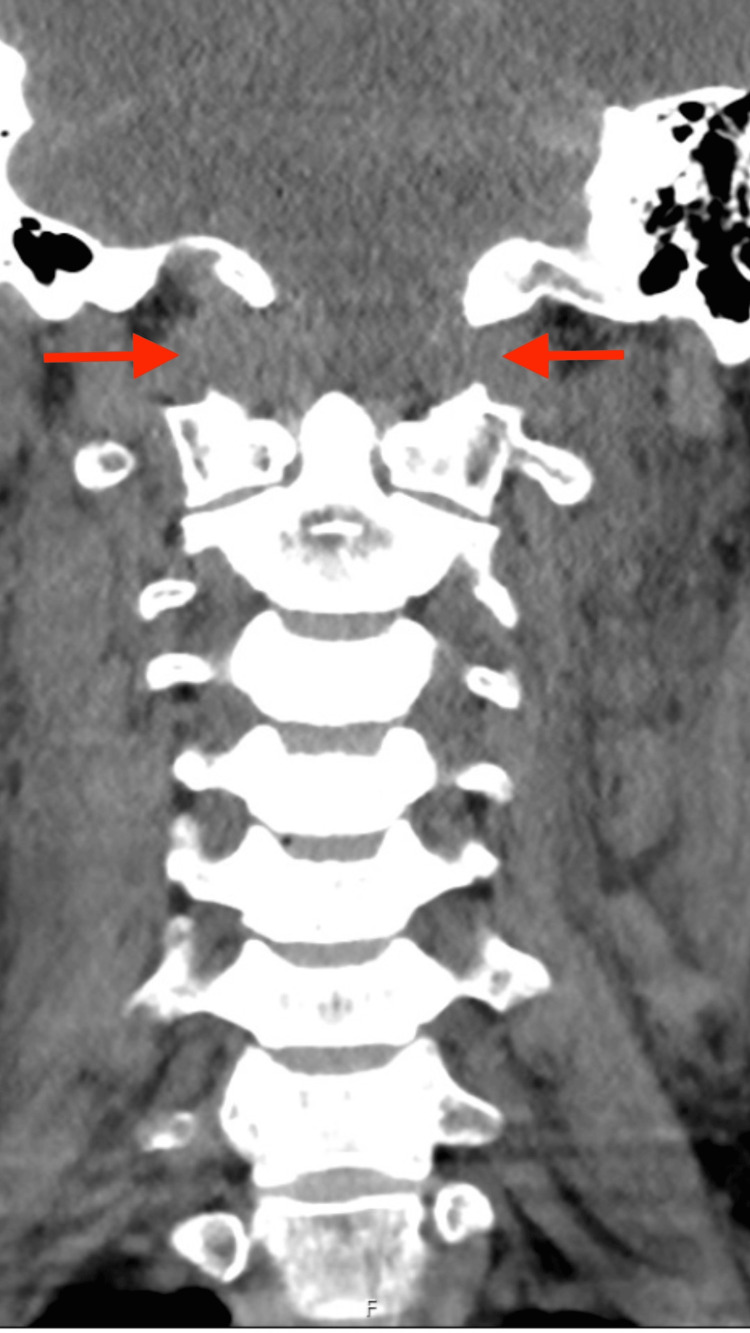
Coronal computed tomography (CT) reconstruction showing widening of the occiput–C1 joint space (arrows), consistent with atlanto-occipital dissociation.
